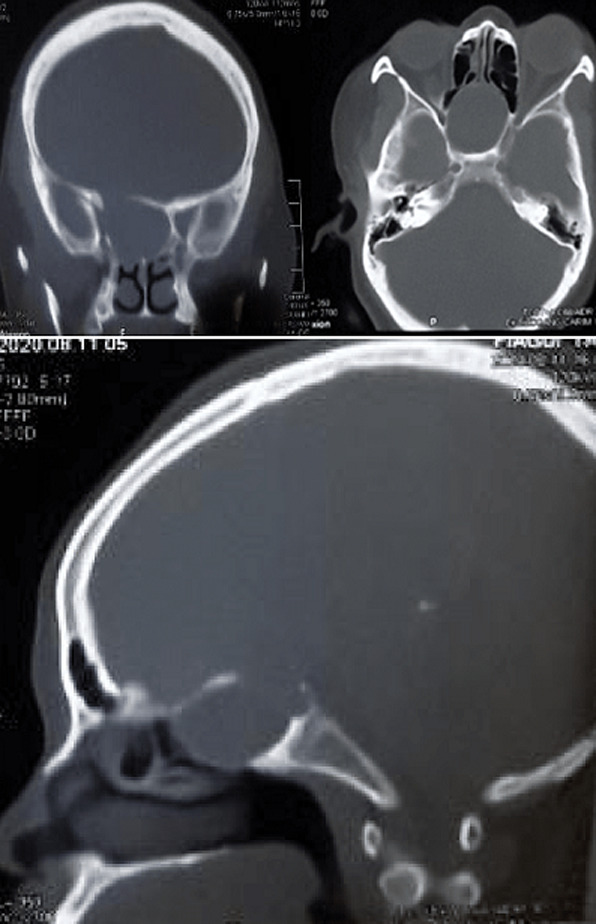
CT scan of the head, showing a roundish isodense lesion occupying the whole sphenoid sinus cavity with erosion of the roof
